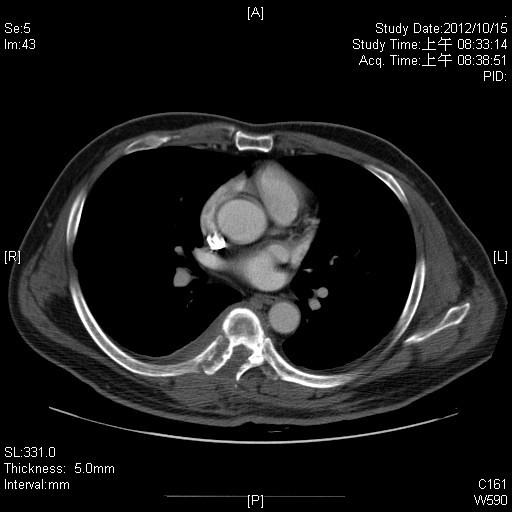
Chest computed tomography scan revealed osteolytic lesions in the right 7th rib with surrounding soft tissue swelling.
